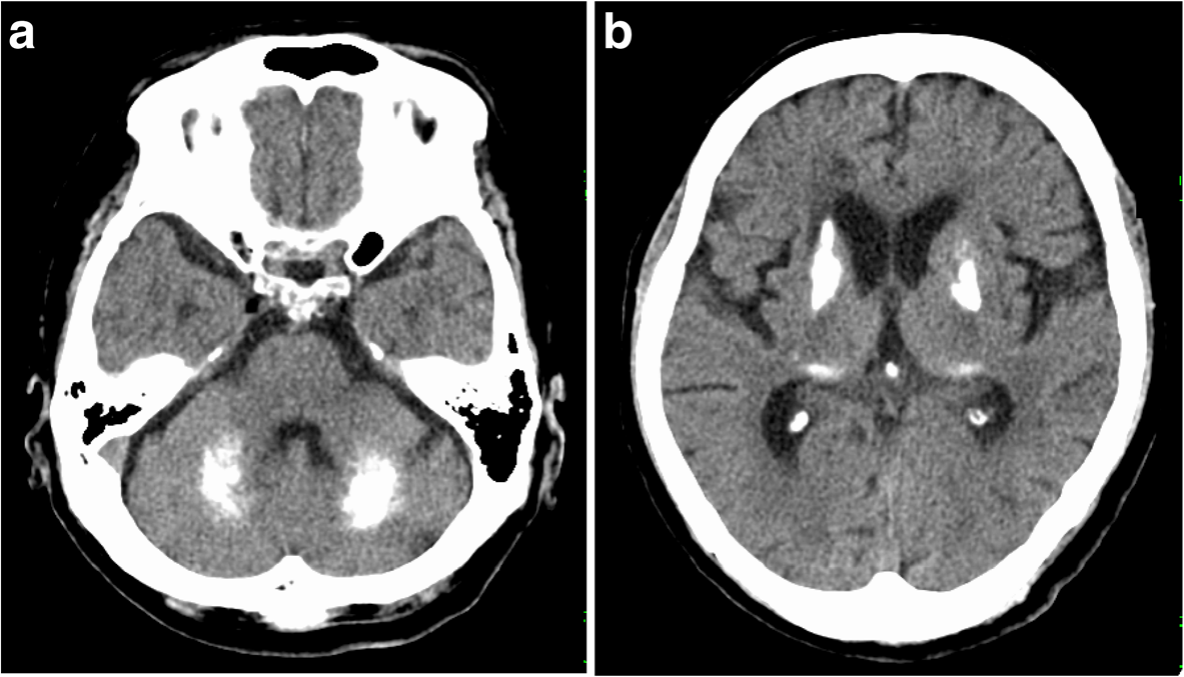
Brain computed tomography (CT). Axial noncontrast CT showing expansive and symmetric high-density lesions involving the cerebellar dentate nuclei (a) and bilateral basal ganglia and thalami (b)
